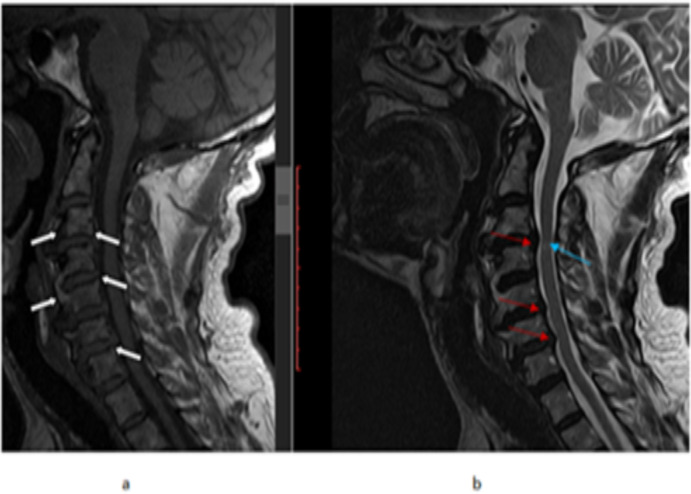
A 55 year old male patient, cervical spine MRI (a) T1W sagittal image showing multiple level anterior and posterior marginal osteophytes (white arrows) (b) T2W sagittal image showing decreased intervertebral disc signal and height from C2-C3 to C6-C7 levels with posterior disc osteophyte complexes at C3-C4, C5-C6 and C6-C7 levels(red arrows). There is also focal spinal cord signal change and decreased caliber at C3-C4 level (blue arrow).
